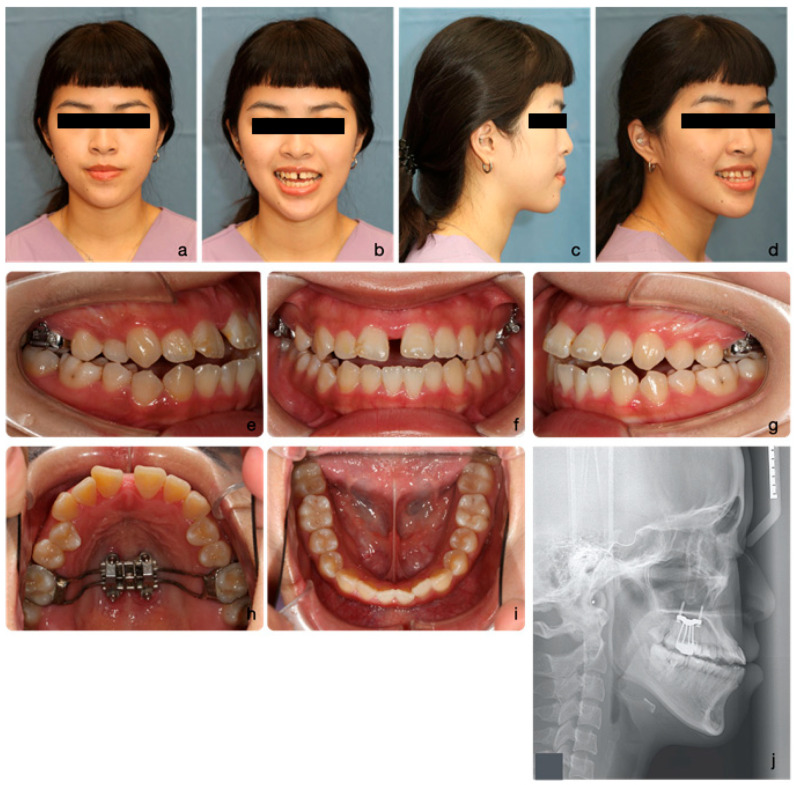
Post-MARPE facial portraits (a–d), intra-oral photos (e–i) and lateral cephalometric radiograph (j). A median diastema was present as a result of mid-palatal suture opening. The mandible underwent backward rotation due to changes in molar occlusion.
